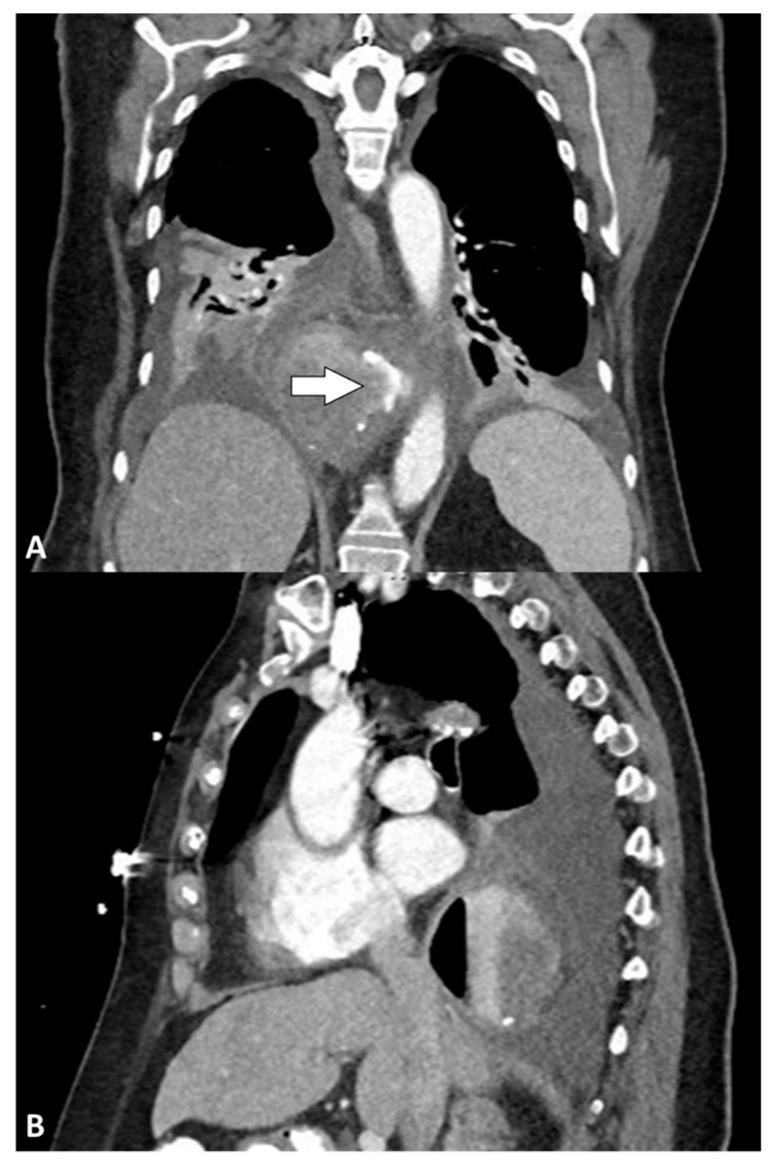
Computed tomography: (A) coronal and (B) sagittal views showing air and fluid collection surrounding the distal esophagus with oral contrast extravasation (arrow).
